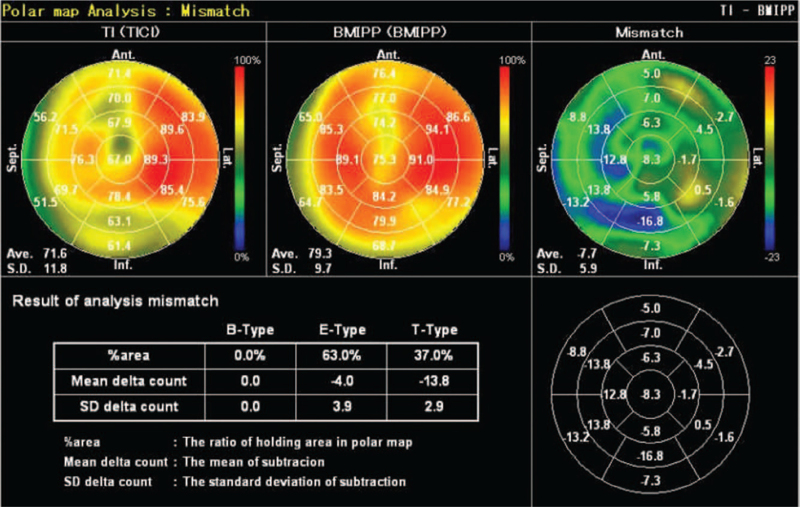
^123^I-BMIPP/^201^TlCl scintigraphy images reveal no mismatch in ^123^I-BMIPP and ^201^TlCl uptake.
